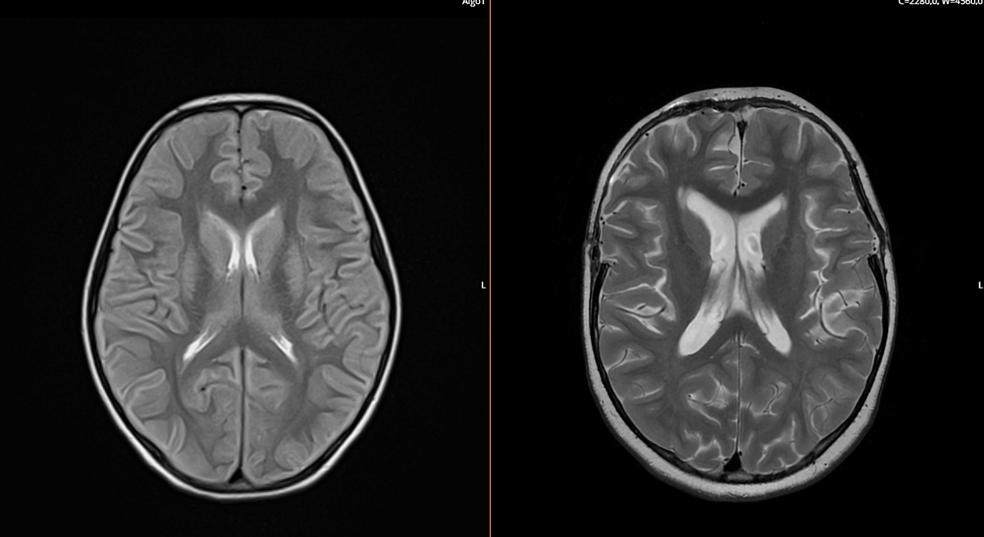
A comparison of the magnetic resonance images on the fourth day of hospitalization (left) and after recovery (right). Note the cytotoxic edema associated with a swollen appearance, resulting in a reduction in the patency of cortical sulci
